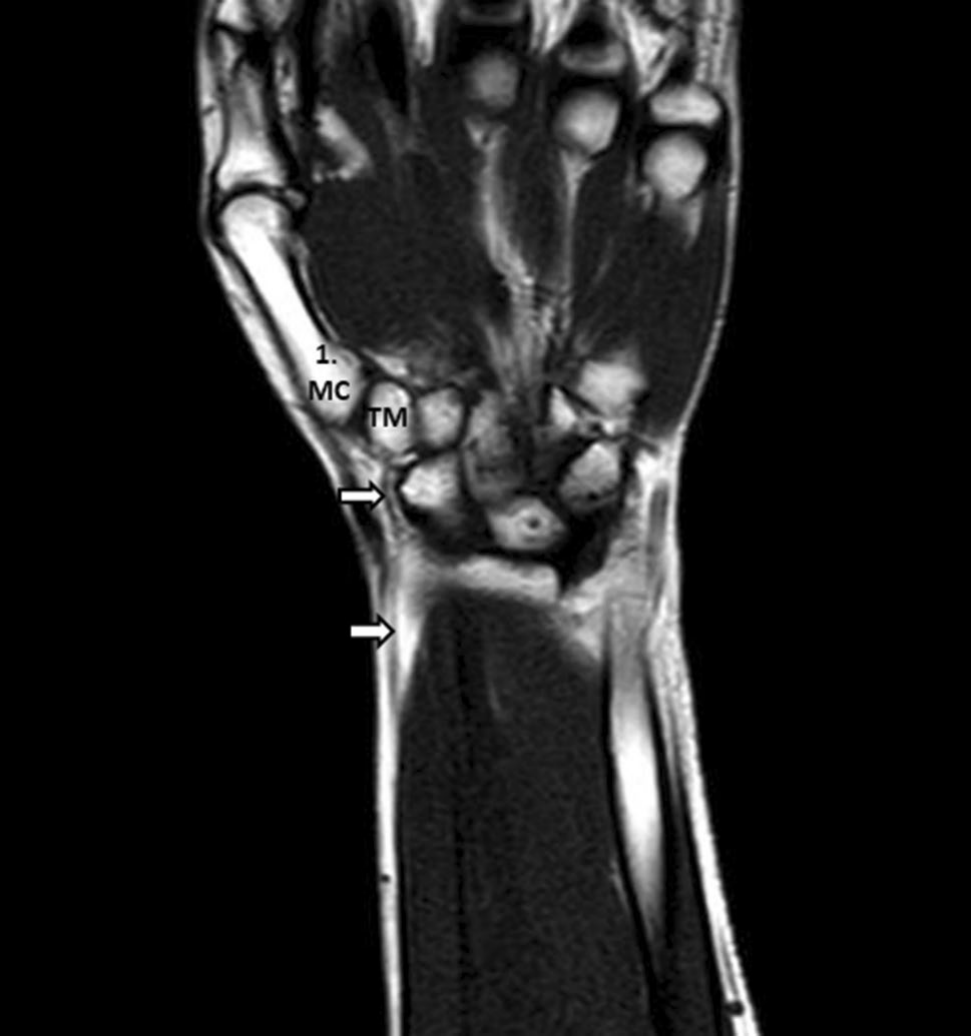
Coronal T1W TSE MR image of the wrist; m. flexor carpi radialis tendon (arrow), os metacarpale (MC), os trapezium (TM).
